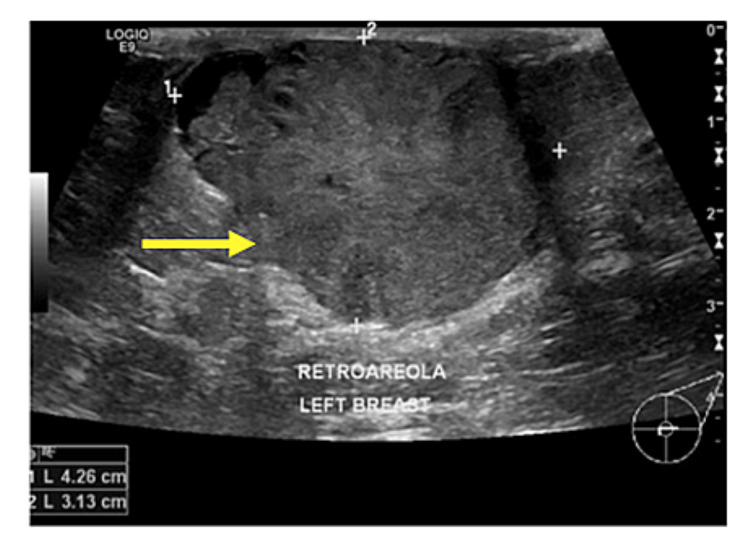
Pathology-proven papilloma presenting as a large retroareolar mass on ultrasound (yellow arrow).
